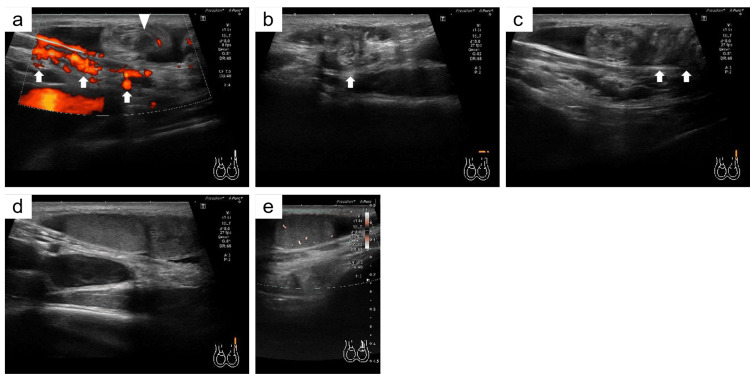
Ultrasonography images a) Arrows show the spermatic cord within the inguinal canal. The triangle shows the spermatic cord above the inguinal canal. b) The spermatic cord is directed toward the body surface (arrow). c) Arrows show multiple twists in the spermatic cord. d) The left testis shows normal echogenicity. e) Power Doppler ultrasound shows preservation of blood flow in the left testis.
